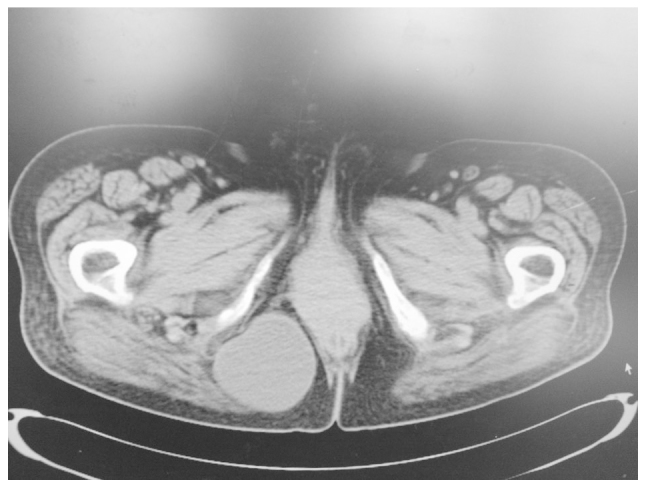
Computed tomography scan showing the location of the tumor beside the anus, and the appreciable affect on its normal structure.
